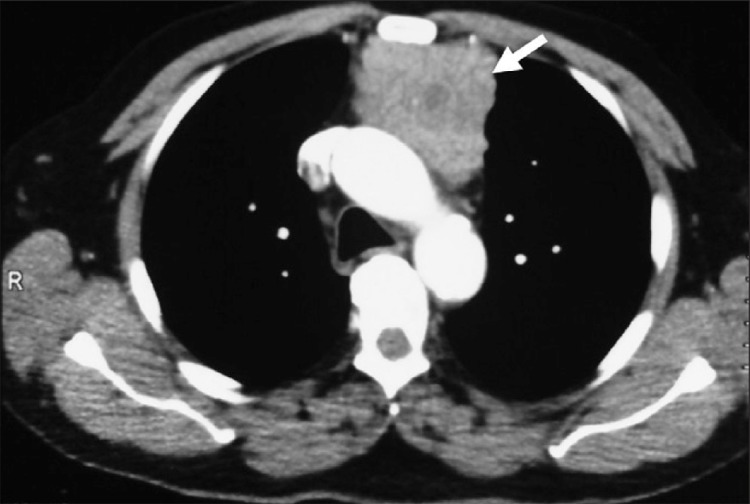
Contrast-enhanced thorax CT shows soft tissue mass in theanterior mediastinum (arrow)
